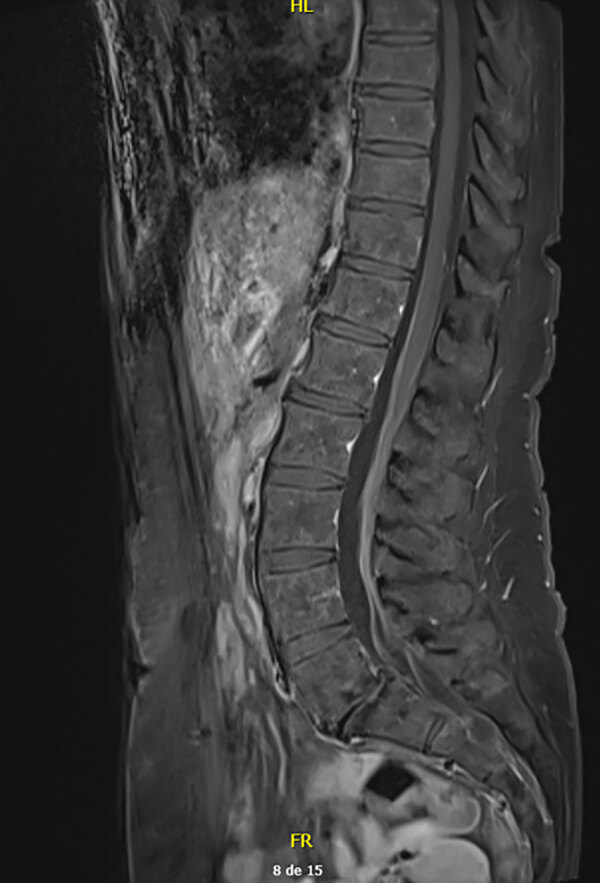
Contrast-enhanced T1-weighted magnetic resonance imaging scans of spine and cauda equina in study of *Borrelia spielmanii*–associated neuroborreliosis in patient receiving rituximab, Belgium. Subtle intradural contrast enhancement was noted after gadolinium injection. No hypertrophy of the cauda equina was found.
